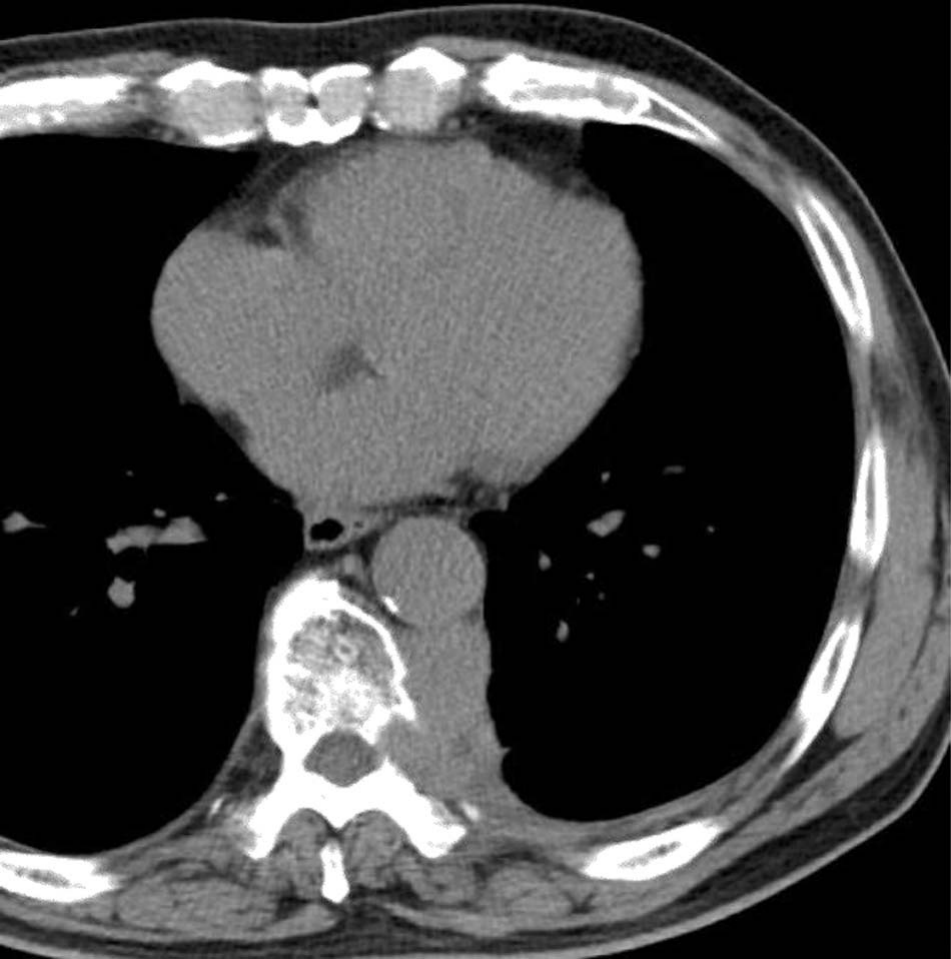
The computed tomographic findings after 6 weeks after radiotherapy.
